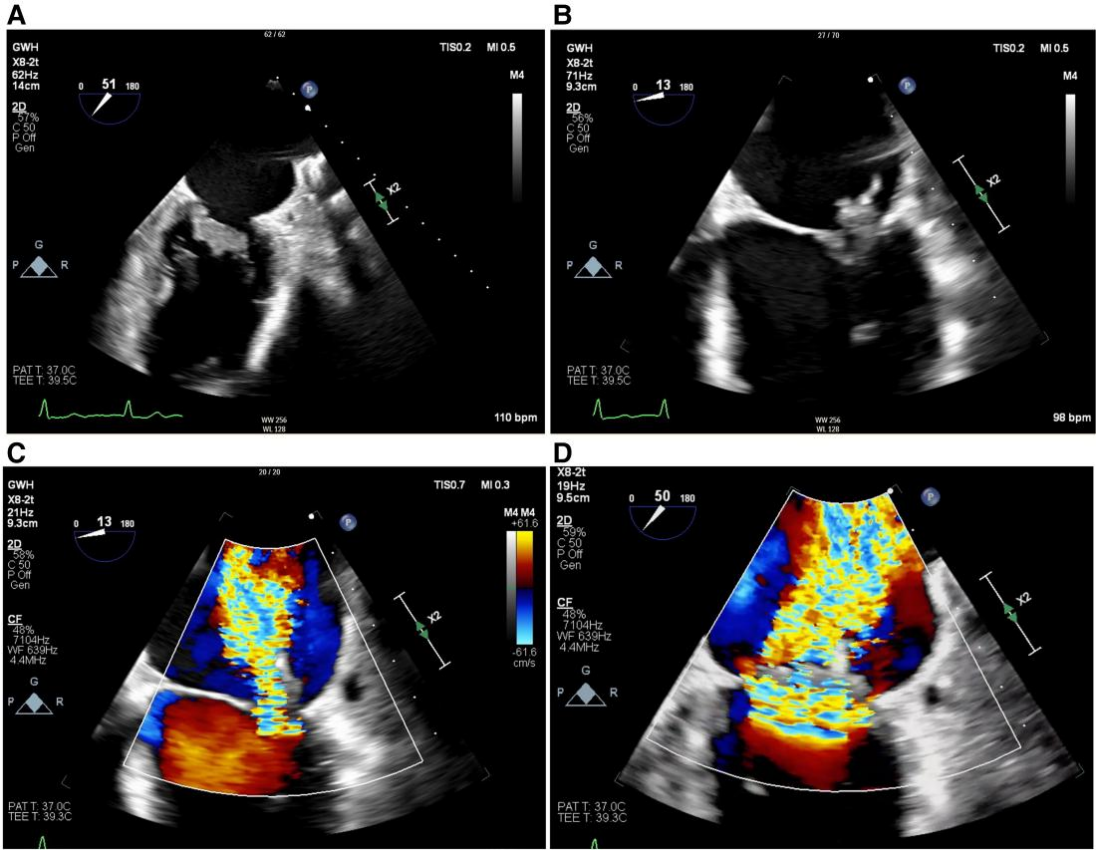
Transoesophageal echocardiogram: (*A*) four-chamber view showing a large mitral valve lesion; (*B*) two-chamber view showing a large mitral valve mass; (*C*) colour flow Doppler of the mitral valve in four-chamber view; and (*D*) in two-chamber view showing a broad jet of mitral regurgitation.
